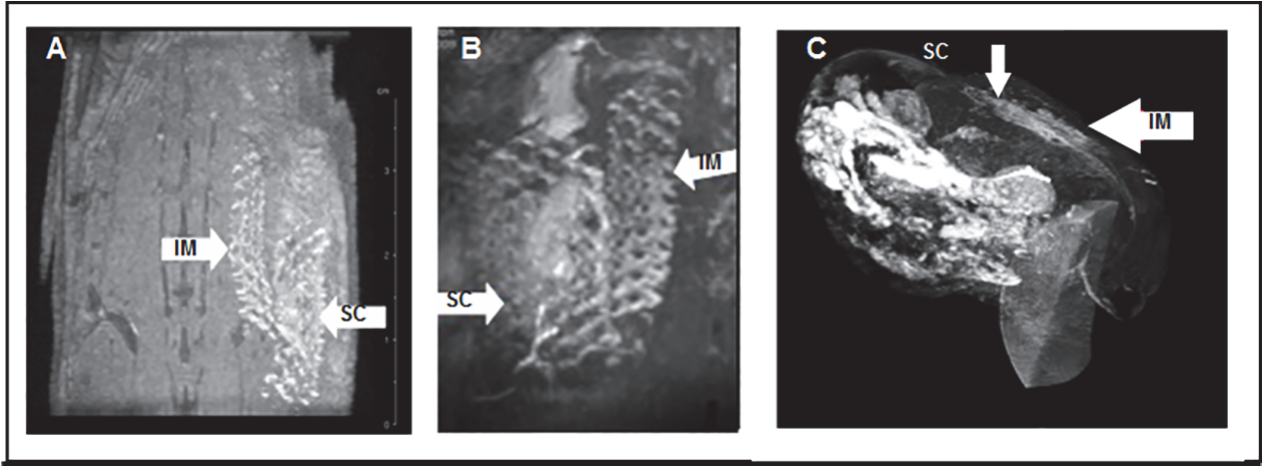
In vivo visualisation of the mesh. (A) Frontal and (B) lateral view of Gd-PMA coated meshes as seen with a 7T MRI device, 3D reconstruction was obtained with OsiriX software. (C) 3D reconstruction of a Gd-PMA coated mesh (dorsal implantation) (3T MRI). Note MRI imaging and 3D reconstruction allow us to distinguish between two meshes (arrows) implanted subcutaneously (SC) and intramuscularly (IM) within few millimetres distance. Scale bars in A and C 1cm, B 5mm.
